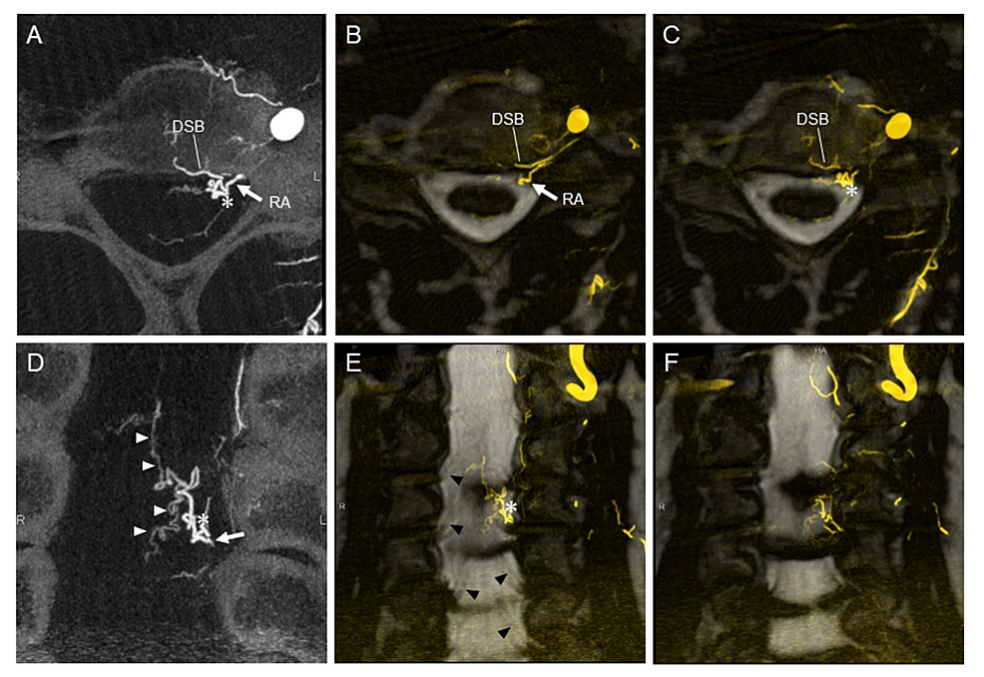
Clinical imaging of de novo RAVF (second lesion) Detailed image analysis shows the de novo RAVF supplied from the radicular artery draining into the ASV (A-F). The shunt is located intradural and on the nerve root. Axial view: (A) 3D-RA MIP image reconstructed from the left VAG, (B, C) MRI fusion images with 3D-RA MIP of the left VAG. Coronal view: (D) 3D-RA MIP image reconstructed from the left VAG, (E, F) MRI fusion images with 3D-RA MIP of the left VAG. White arrowheads show draining veins, black arrowheads show the spinal nerve roots, and an asterisk indicates the shunt point. 3D-RA, three-dimensional rotational angiography; ASV, anterior spinal vein. AVF, arteriovenous fistula; DSB, dorsal somatic branch; MIP, maximum intensity projection; MRI, magnetic resonance image; RA, radicular artery; VAG vertebral artery angiography
